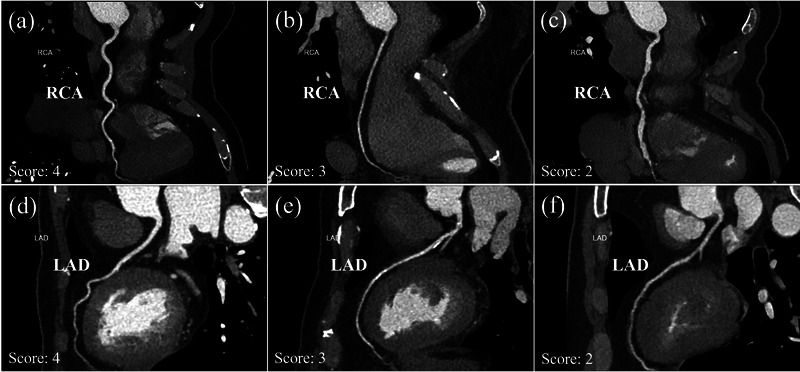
Coronary CT angiography: examples of different levels of image quality. Right coronary artery (RCA) with score of 2−4 (a‐c); Left anterior descending (LAD) coronary artery with score of 2−4 (d‐f).
